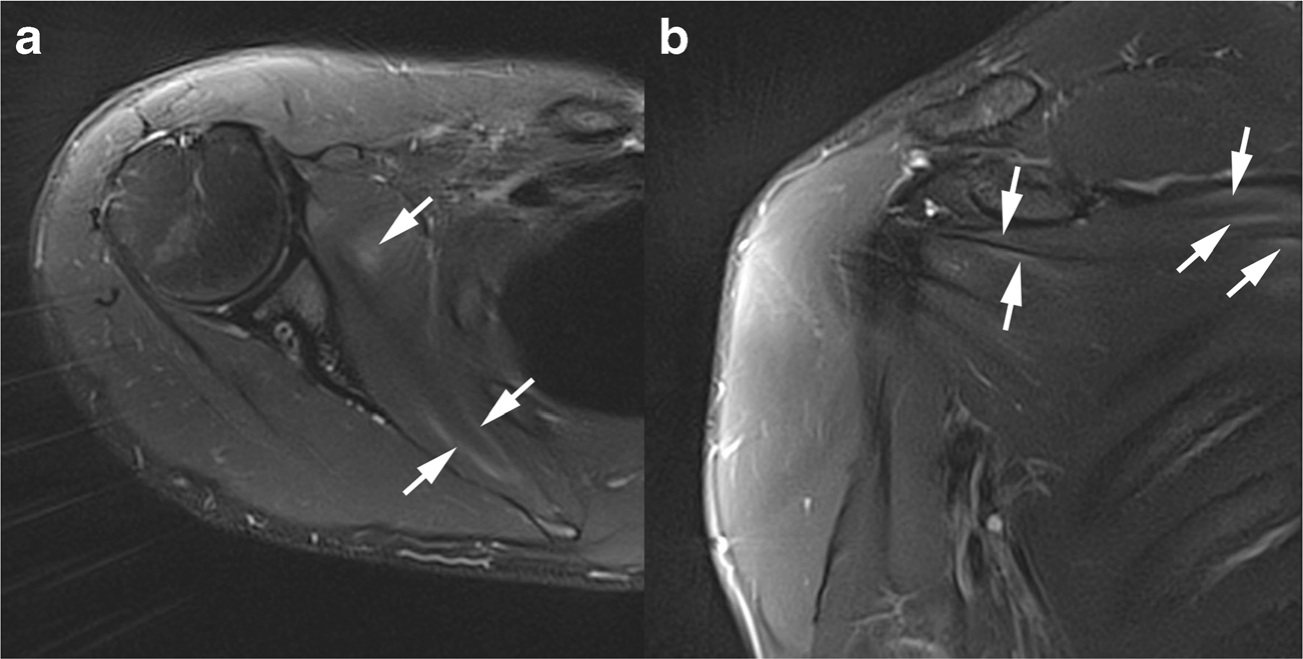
Right shoulder MRI of a 21-year-old military serviceman with sharp pain of the right shoulder, occurring after military training. Axial (a) and coronal oblique (b) FS DP weighted images. Mottled and linear high signal areas in the SSC muscle mostly at the myotendinous junctions (white arrows) are secondary to intrinsic muscle lesions
